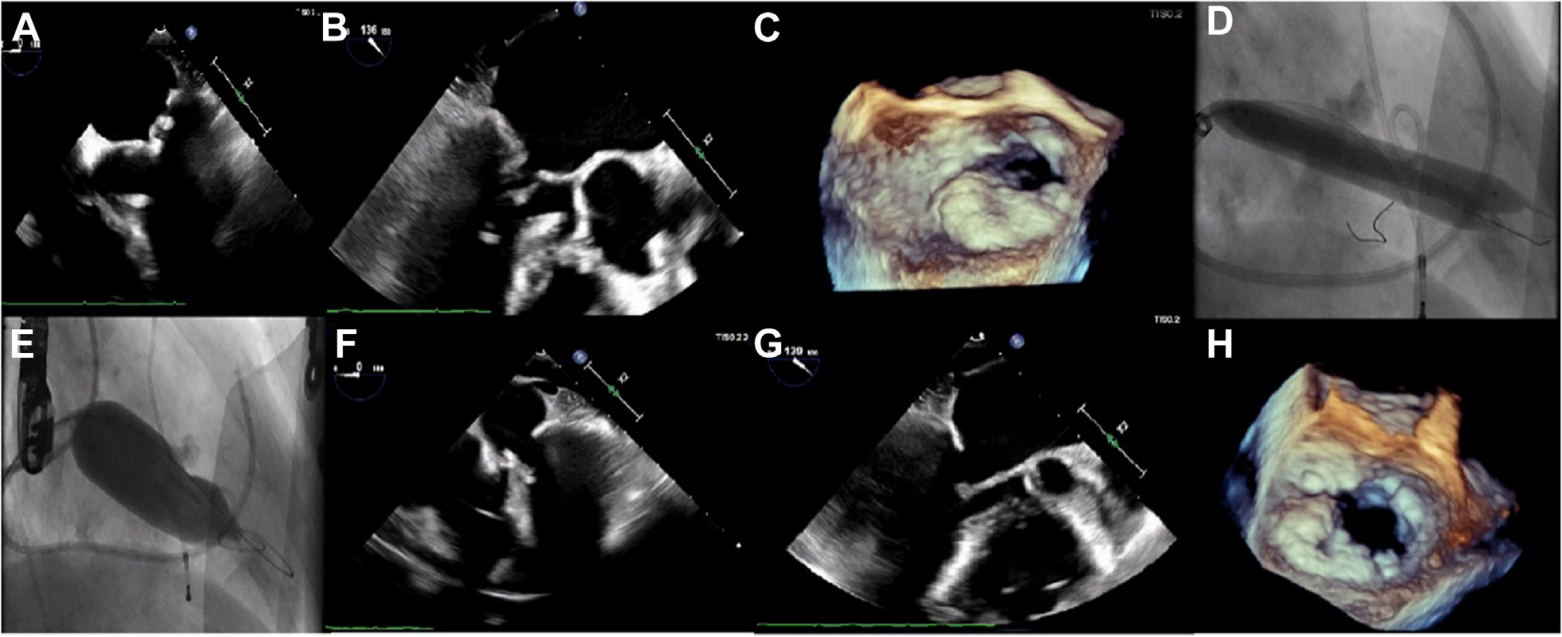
(A, B,C) Preprocedure transesophageal echocardiogram of severely calcified stenotic mitral valve with significant posterior leaflet calcification and decreased mobility (Supplemental Video 1). (D) Mitral valvuloplasty with 2 simultaneous 8.00 × 60-mm Shockwave lithotripsy balloons. (E) Mitral valvuloplasty with 25 × 40-mm True Dilation balloon (Supplemental Video 2). (F, G, H) Postprocedure transesophageal echocardiogram showing improvement in mitral valve area and leaflet mobility (Supplemental Video 3).
